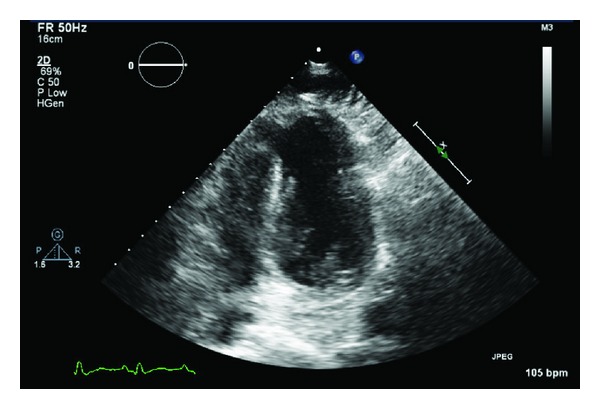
Transthoracic echocardiogram demonstrating ventricular septal defect.
